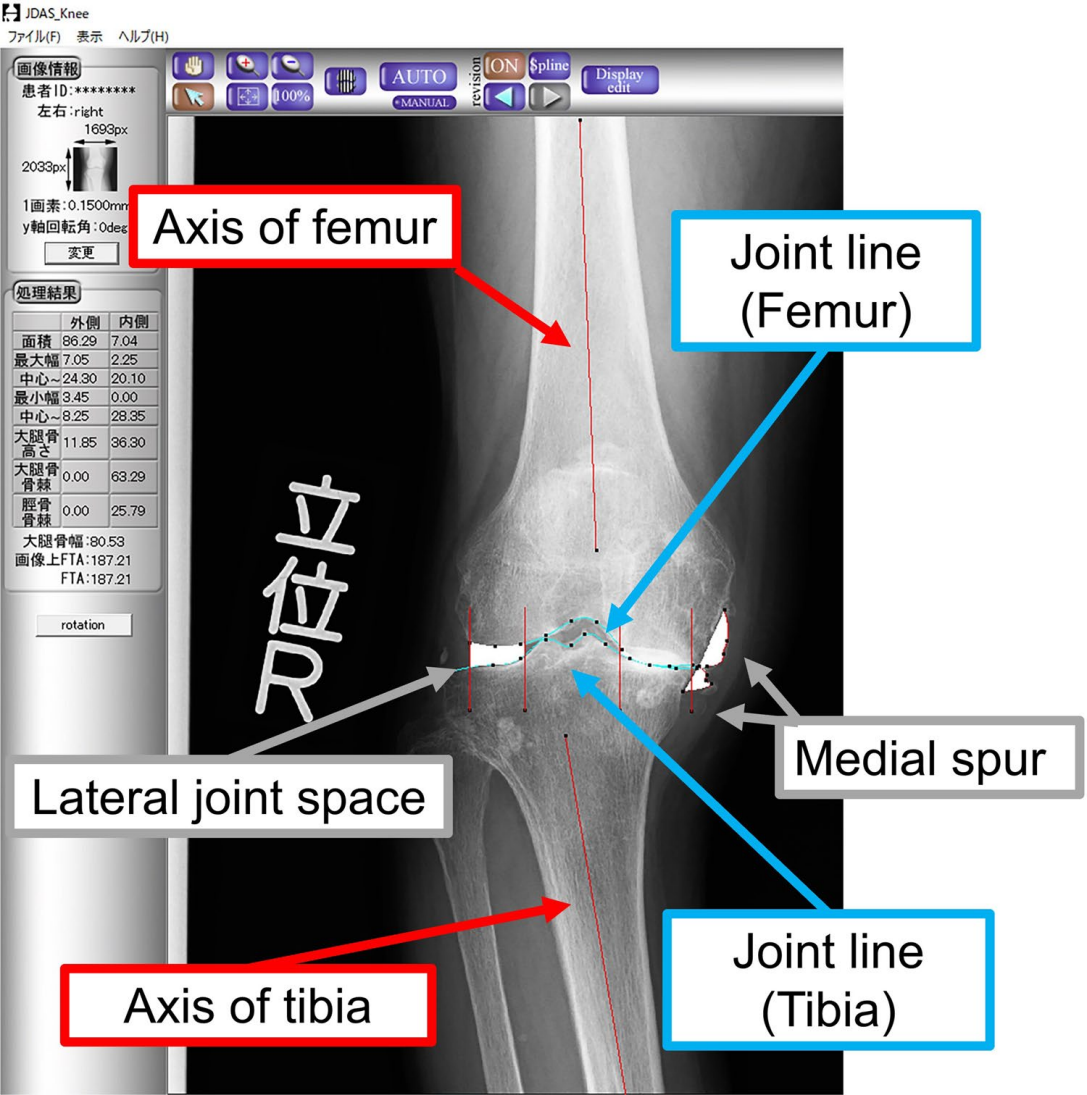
Automatic measurements of the radiographic parameters performed by knee osteoarthritis computer-aided diagnosis (KOACAD) software
